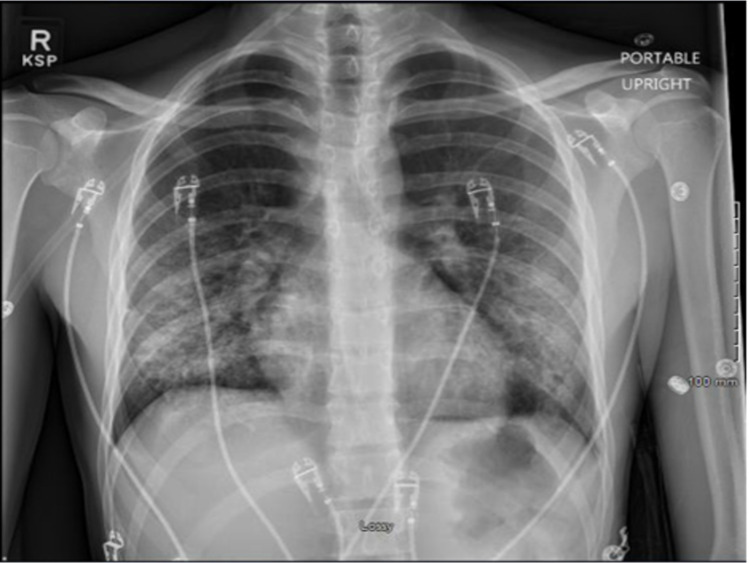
Chest X-ray after methylprednisolone treatment.
